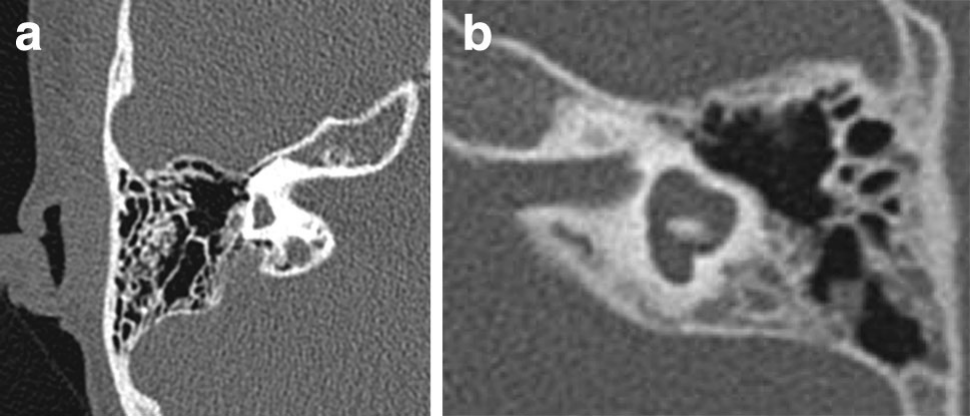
Range of abnormalities of the vestibular system seen in axial CT images. a Aplasia of the semicircular canals in a right ear. b Dysplasia of the vestibule and semicircular canals in a left ear, with a malformed vestibule, shortened and dilated horizontal semicircular canal with small bony island, incomplete formation and dilatation of the posterior semicircular canal
